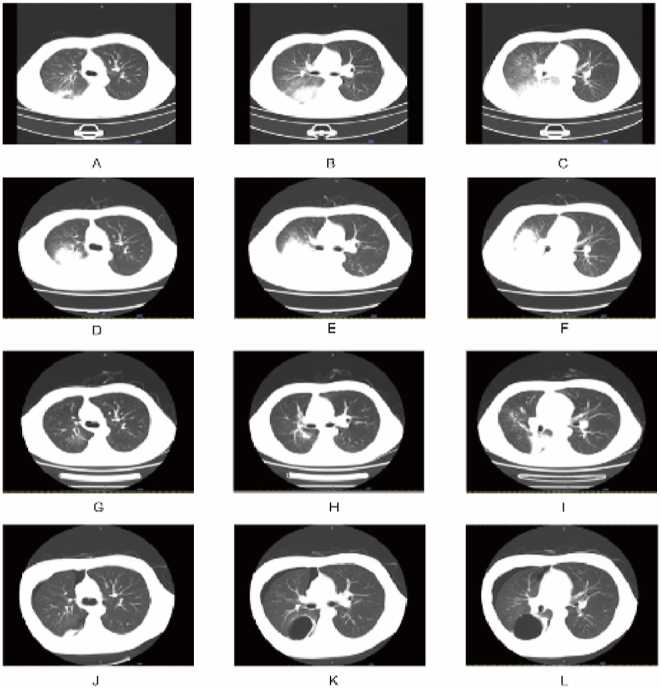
Chest computed tomography (CT) images of an adolescent patient with macrolide-unresponsive *Mycoplasma penumoniae* pneumonia. (A–C) Chest CT on hospital Day 1, showing that patchy consolidation of the right lung, significant in the right lower lung. (D–F) Chest CT on hospital Day 9, showing that the original consolidation in the right lung had become enlarged and new patchy consolidations had appeared in the bilateral lower lobes. (G–I) Chest CT on hospital Day 17, showing significant resolution of the bilateral lung lesions. (J–L) Chest CT on the 2nd day of readmission, showing a right pneumothorax with intrapulmonary bullae.
